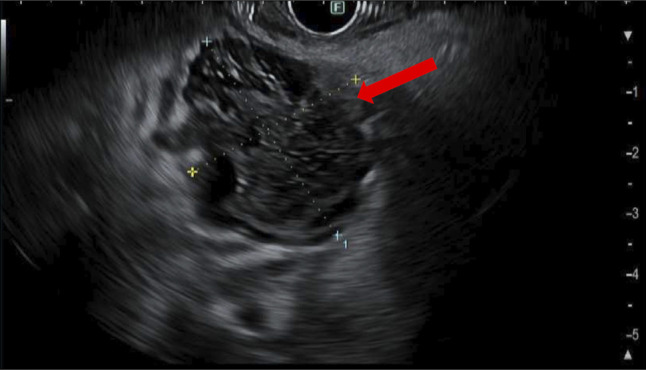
Endoscopic ultrasound showing a 38 × 31-mm peripancreatic fluid collection.
